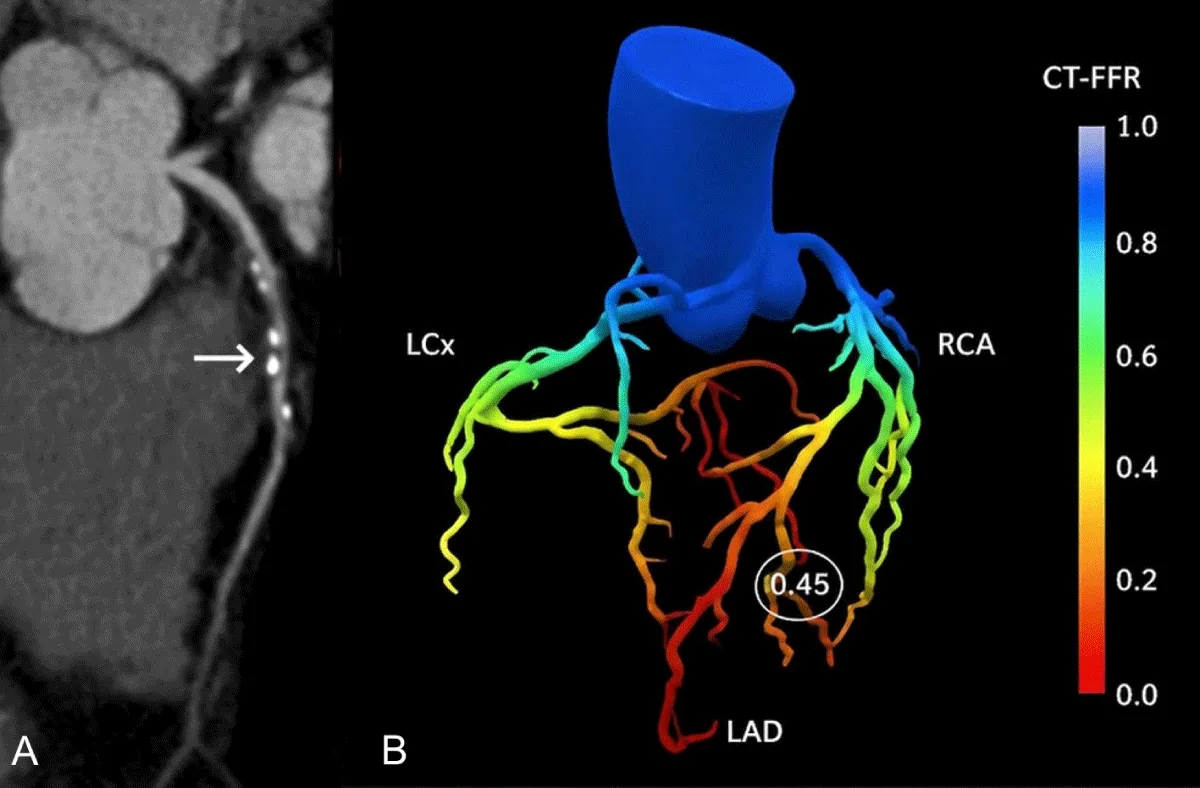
Representative case of CT-derived fractional flow reserve (CT-FFR) analysis. (A) Curved multiplanar reconstruction of coronary CT angiography shows a mixed plaque causing intermediate stenosis in the mid-left anterior descending artery (LAD) (arrow). (B) Three-dimensional CT-FFR model demonstrates a hemodynamically significant lesion in the mid-LAD with a CT-FFR value of 0.45 (circled), while other coronary segments show higher CT-FFR values.
